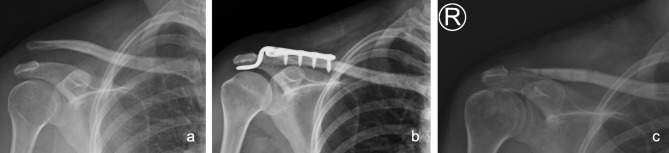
A 49 years old female presented with right shoulder distal clavicle osteolysis after hook plate fixation. (a) Preoperative radiography. (b) Status post open reduction and fixation with hook plate. (c) Distal clavicle osteolysis noted at postoperative 1-year follow up
